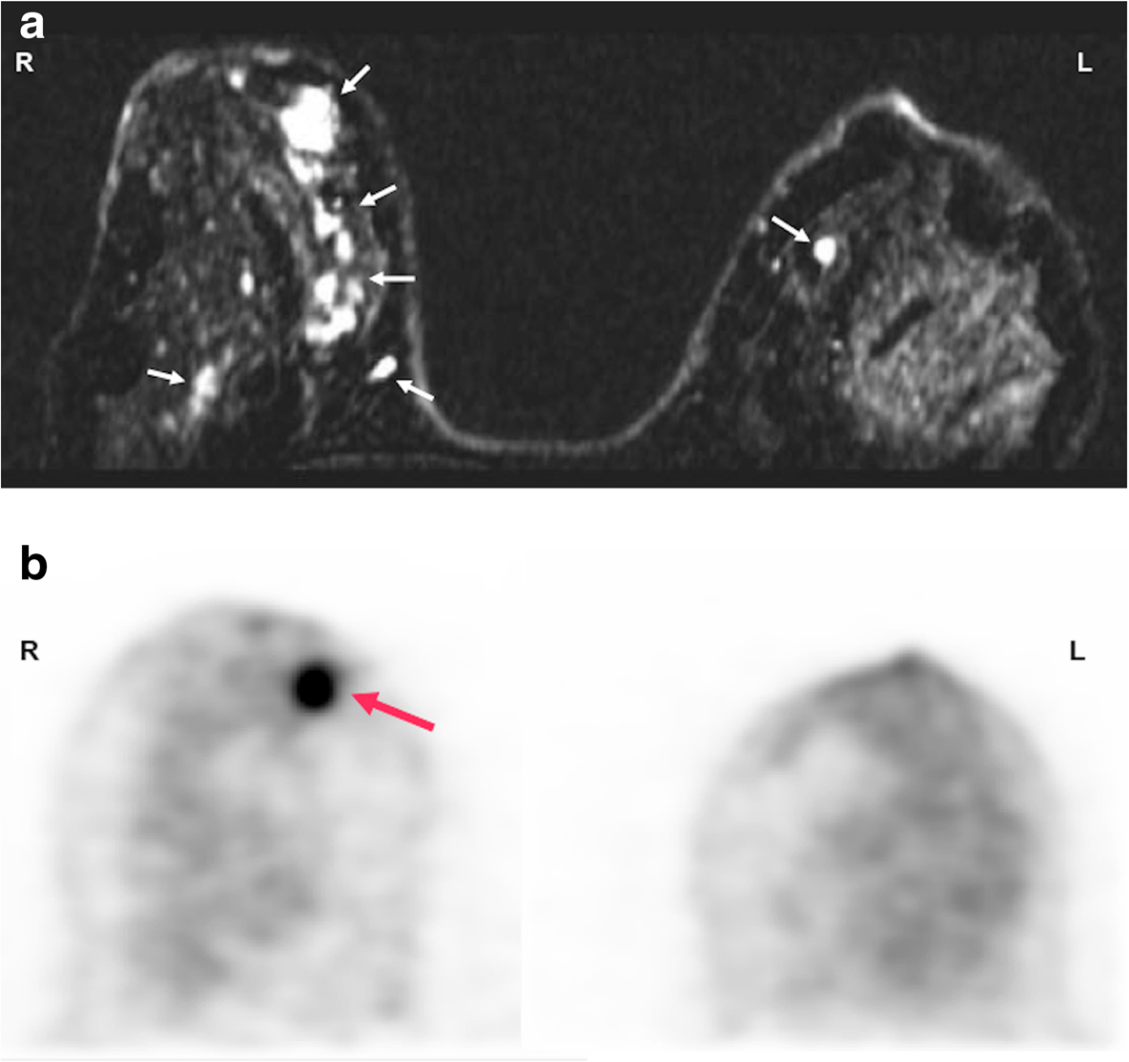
A 48-year-old woman with breast cancer. a Axial contrast-enhanced T1-weighted MR image of breasts performed during last week of menstrual cycle showed bilateral multiple nodular areas of enhancement in both mammary glands (white and red arrows). b A dedicated FDG-PET exam only depicted a nodular mass (red arrow) with increased FDG uptake in the right breast, corresponding to a invasive ductal carcinoma
